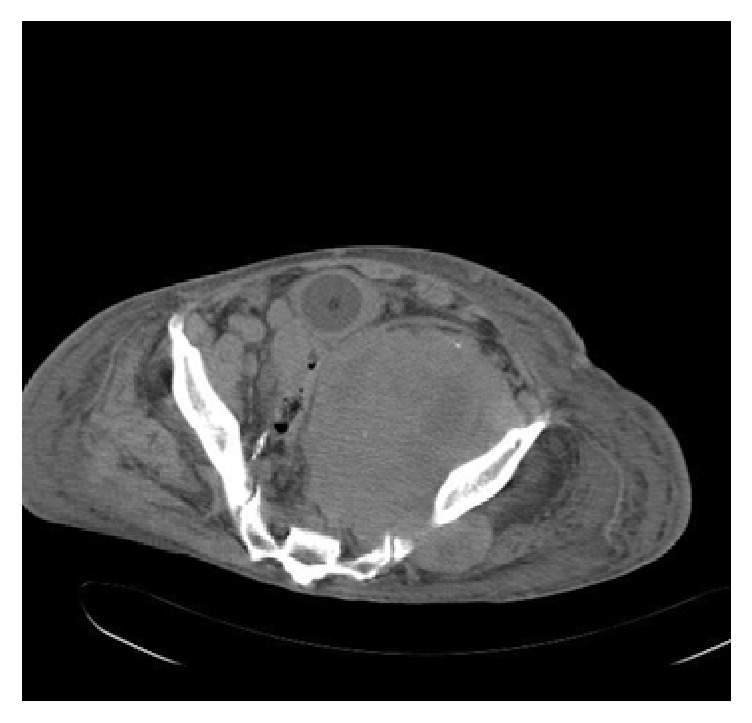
CT of abdomen and pelvis shows the retroperitoneal masses.
